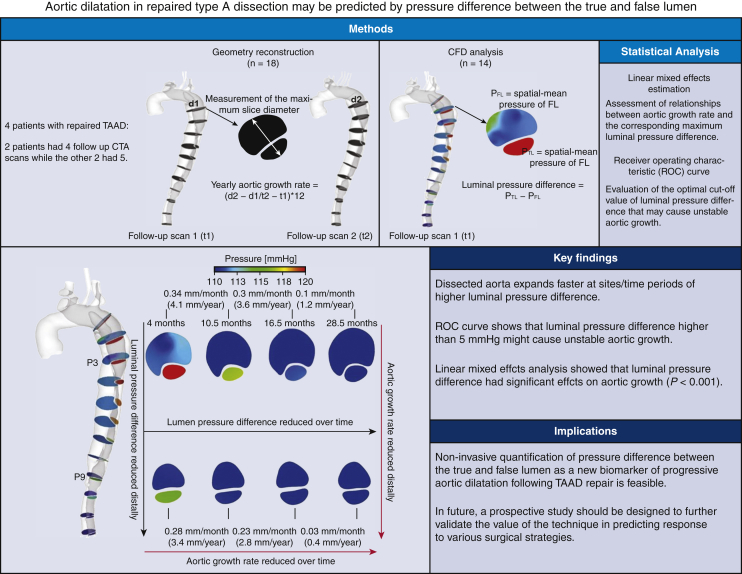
Four patients with surgically repaired type A aortic dissection (*TAAD*) with multiple follow-up computed tomography angiography (*CTA*) images were included in this study. Despite the small number of patients, 18 dissection geometries were reconstructed by making use of all follow-up CTA images, after which the yearly aortic growth rate (mm/year) was evaluated among each cross-sectional plane at every follow-up scan. Computational flow analysis was performed for 14 of 18 geometries, because geometries reconstructed from the last CTA scans were served as comparative purpose only. A key hemodynamic factor, namely, the pressure difference between the true and false lumen, was evaluated at the same cross-sectional planes. The relationship between aortic growth rate and the corresponding luminal pressure difference was then assessed using a linear mixed effects model. Results of the study revealed that luminal pressure difference had a significant effect on aortic growth, suggesting its role as a predictor of aortic dilatation in patients with repaired TAAD. Moreover, a receiver operating characteristic curve shows that a maximum pressure difference of 5 mm Hg (or greater) might cause unstable growth (yearly aortic growth rate >2.9 mm). *CFD*, Computational fluid dynamic; *TL*, true lumen; *FL*, false lumen.
